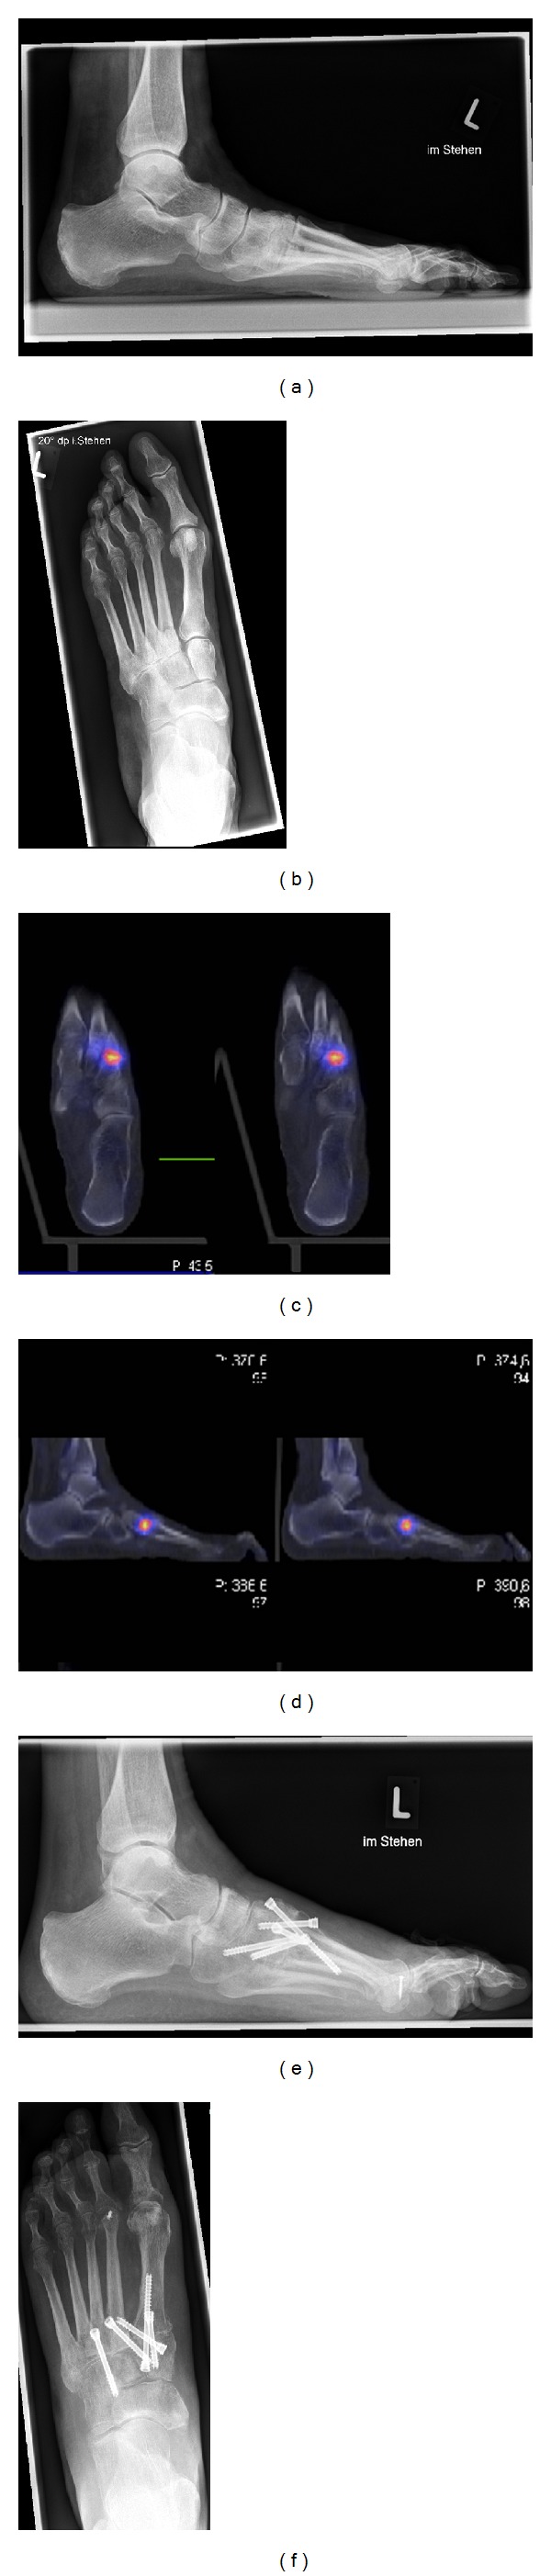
(a) and (b) preoperative plain radiographs; (c) and (d) SPECT-CT; (e) and (f) postoperative plain radiographs. A 63-year-old woman with pes plano-valgus deformity described pain in the whole foot for many years. A conservative treatment with insoles and physiotherapy failed. After clinical examination and plane radiographs subtalar arthritis was the assumed diagnosis. Because of diagnostic uncertainty a SPECT-CT was performed resulting in an active arthritis of TMT II and III and arthritis of TMT-I. After fusion of TMT I-III the patient described pain relief five months after the operation.
